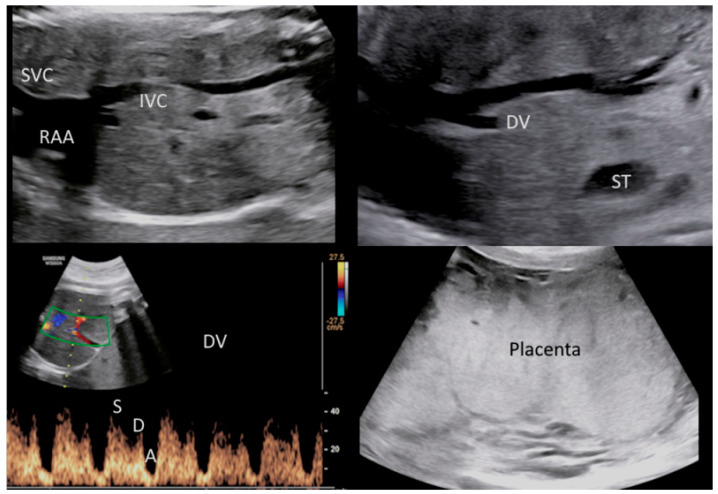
Doppler ultrasound revealed abnormal venous flow of the ductus venosus (DV) with a PI: 1.25. These findings were suspicious for metabolic, infective and/or hypoxic-ischemic events. (Legend: A: atrial contraction; D: diastole; DV: ductus venosus; IVC: inferior vena cava; PI: pulsatility index; RAA: right atrium appendage; ST: stomach; SVC: superior vena cava; S: systole). Ultrasound also documented a placentomegaly.
